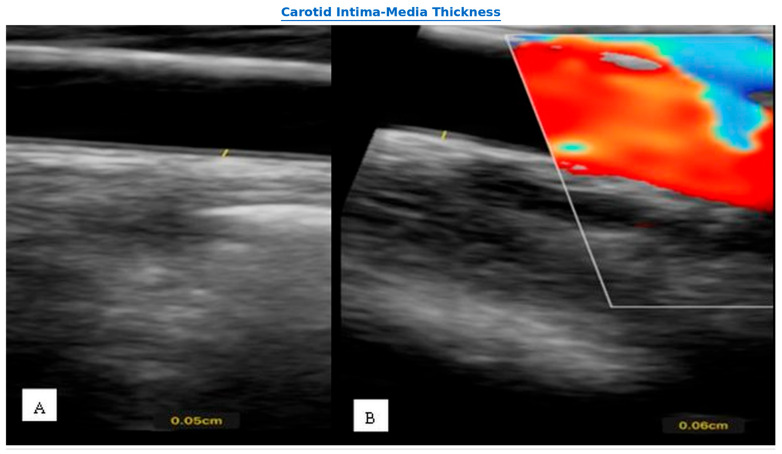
B-mode (grayscale) ultrasound image of the common carotid artery (CCA), showing a Carotid Intima-Media Thickness (CIMT) measurement. The yellow vertical measurement marker visible in the images is placed on the posterior (far) wall of the CCA ((A): 0.05 cm, female; (B): 0.06 cm, male).
